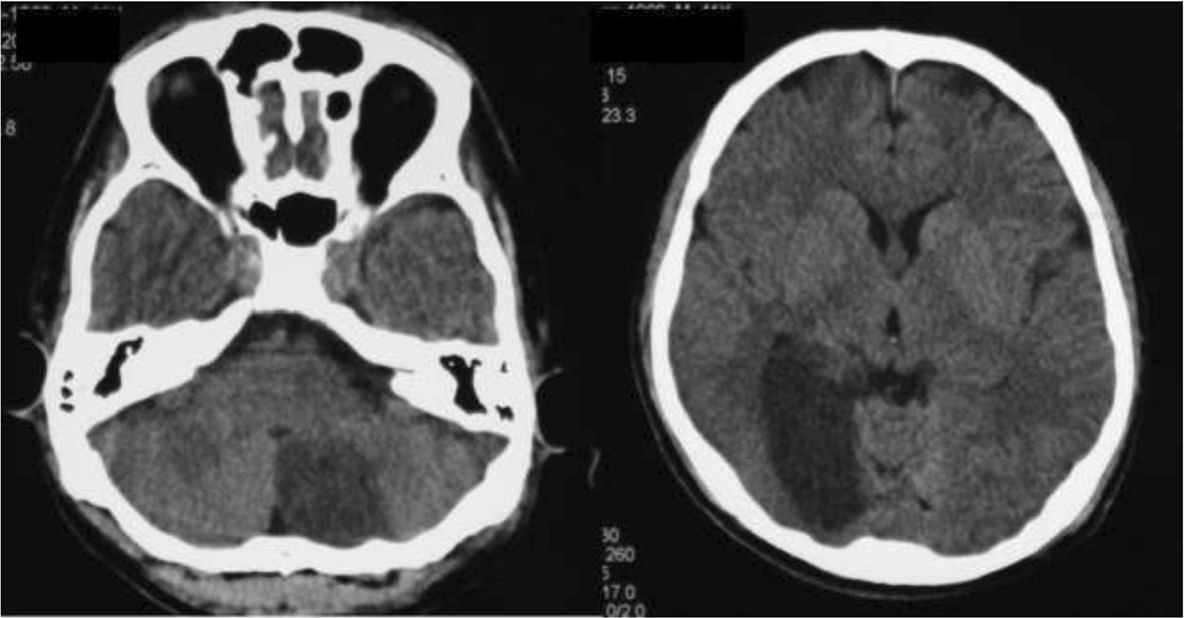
Postoperative brain computed tomography.
